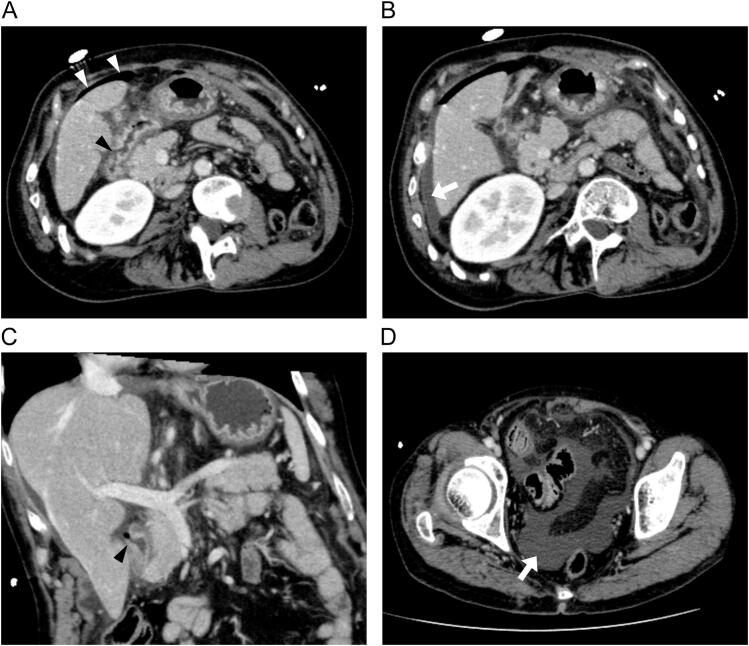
Abdominal contrast-enhanced CT revealed a discontinuity in the duodenal wall (black arrowhead), free gas on the surface of the liver (white arrowhead), and a small amount of ascites (white arrow) (panels A, B, C). A moderate amount of ascites (white arrow) was also observed in the Douglas fossa (panel D).
